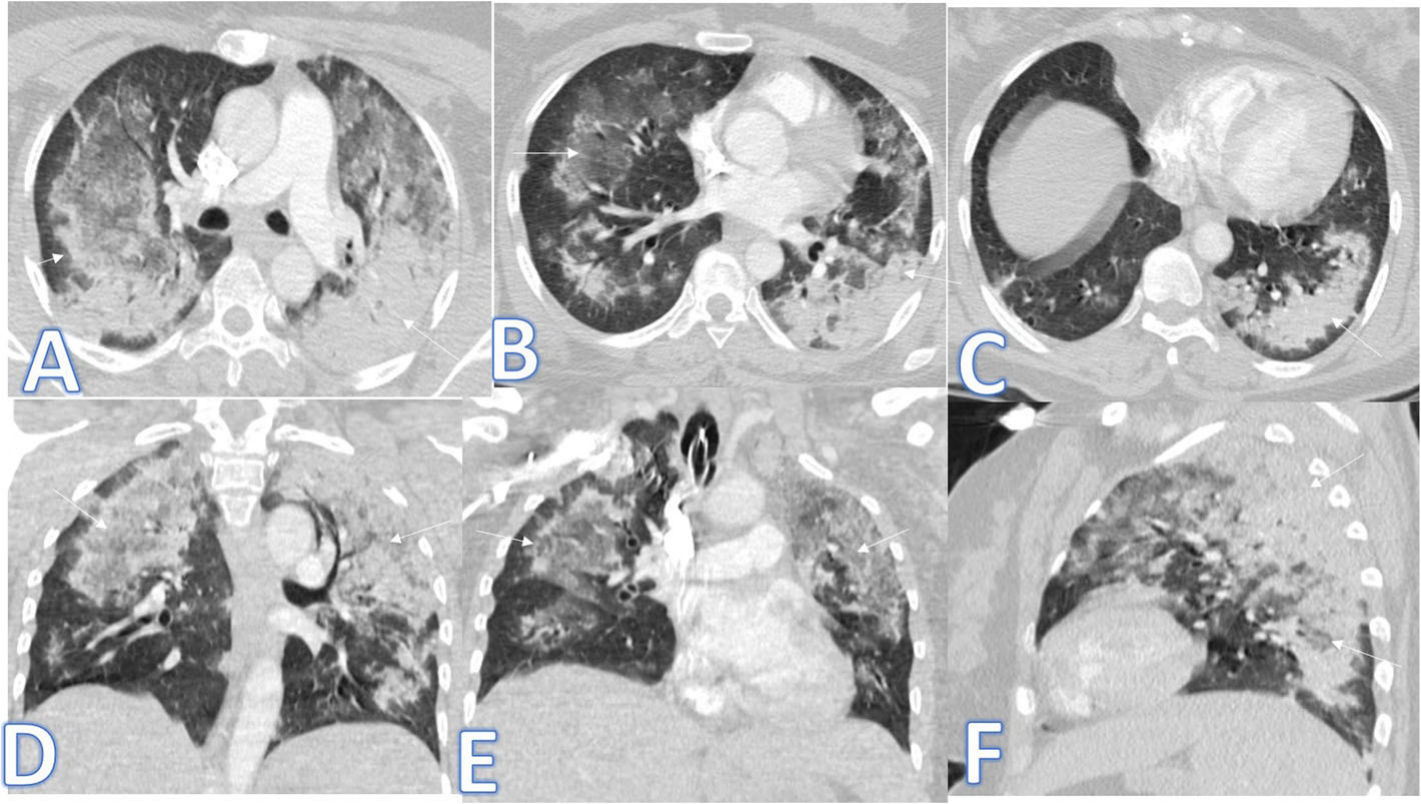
A 46-year-old female patient with SLE, presented with hemoptysis. CT showed bilateral confluent consolidative patches with GGO (arrows) more predominant in the upper lobes with subpleural and costophrenic angle sparing. The diagnosis was diffuse alveolar hemorrhage
